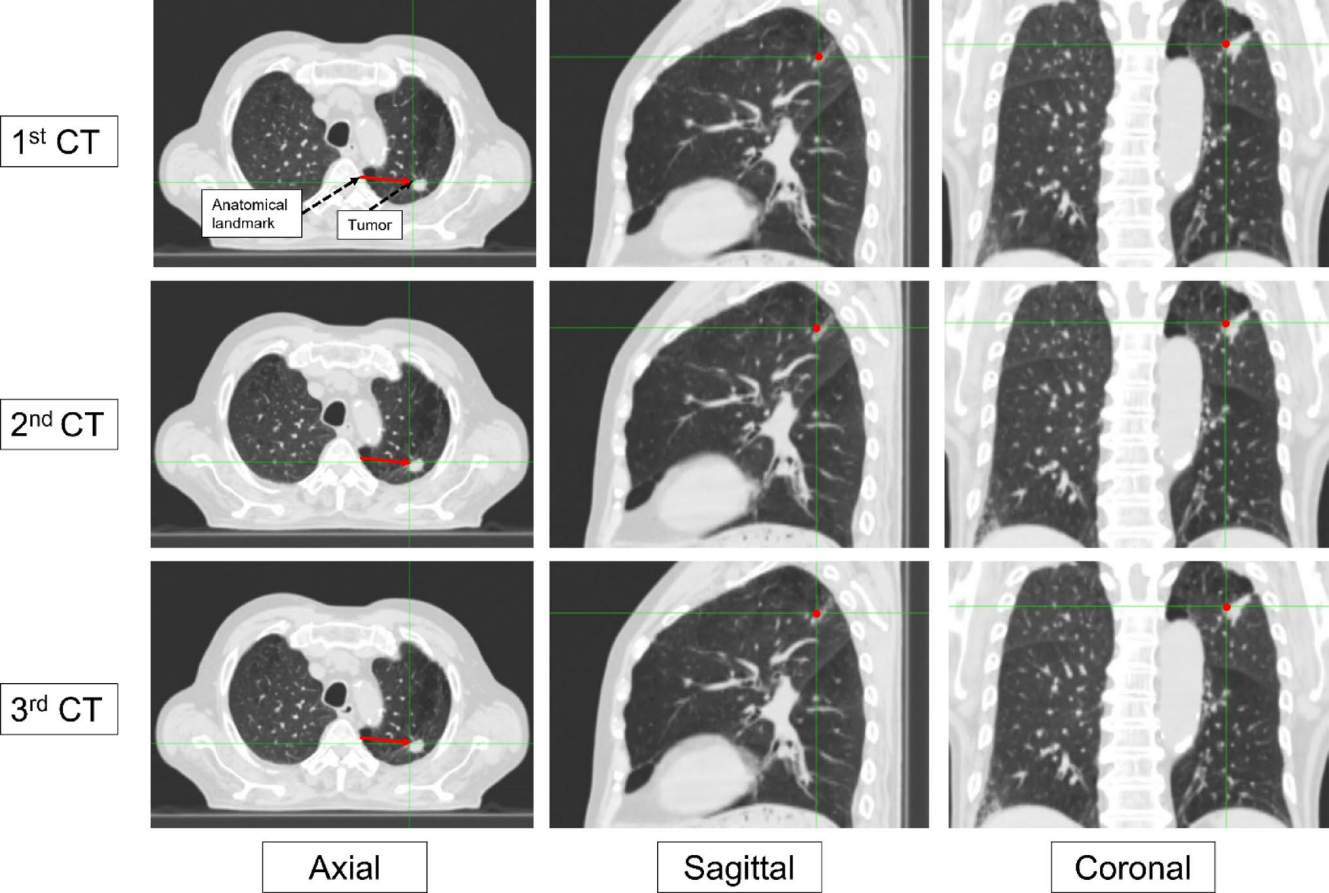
Three repeated CT images of a typical case are shown. From left to right, axial, sagittal, and coronal views are presented. In the axial view, the red arrow indicates the distance from an anatomical landmark to the tumor, while in the sagittal and coronal views, the red point denotes the same anatomical landmark relative to the tumor
